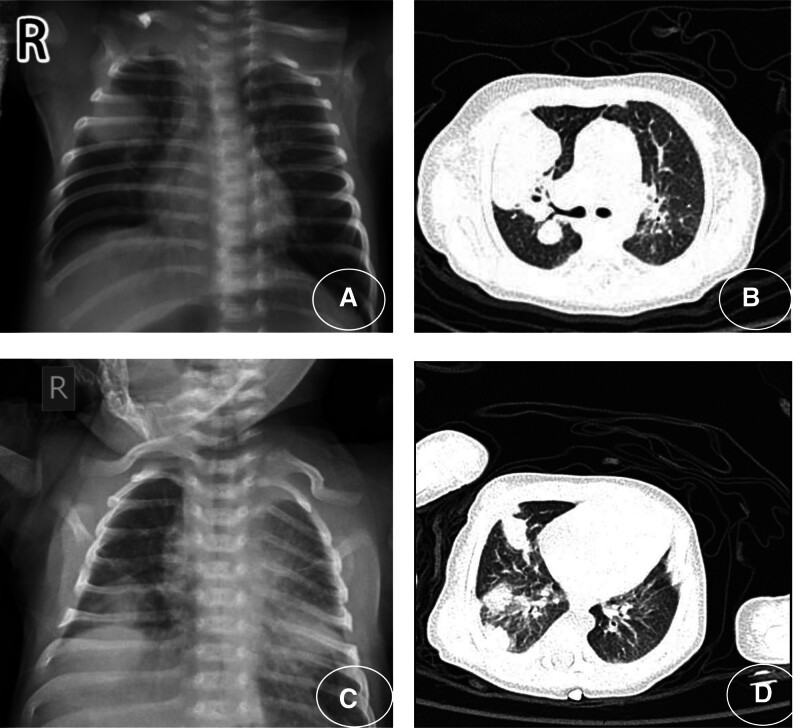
Pulmonary radiology (lung X-ray and computed tomography): case 2 (A and B) and case 3 (C and D).
